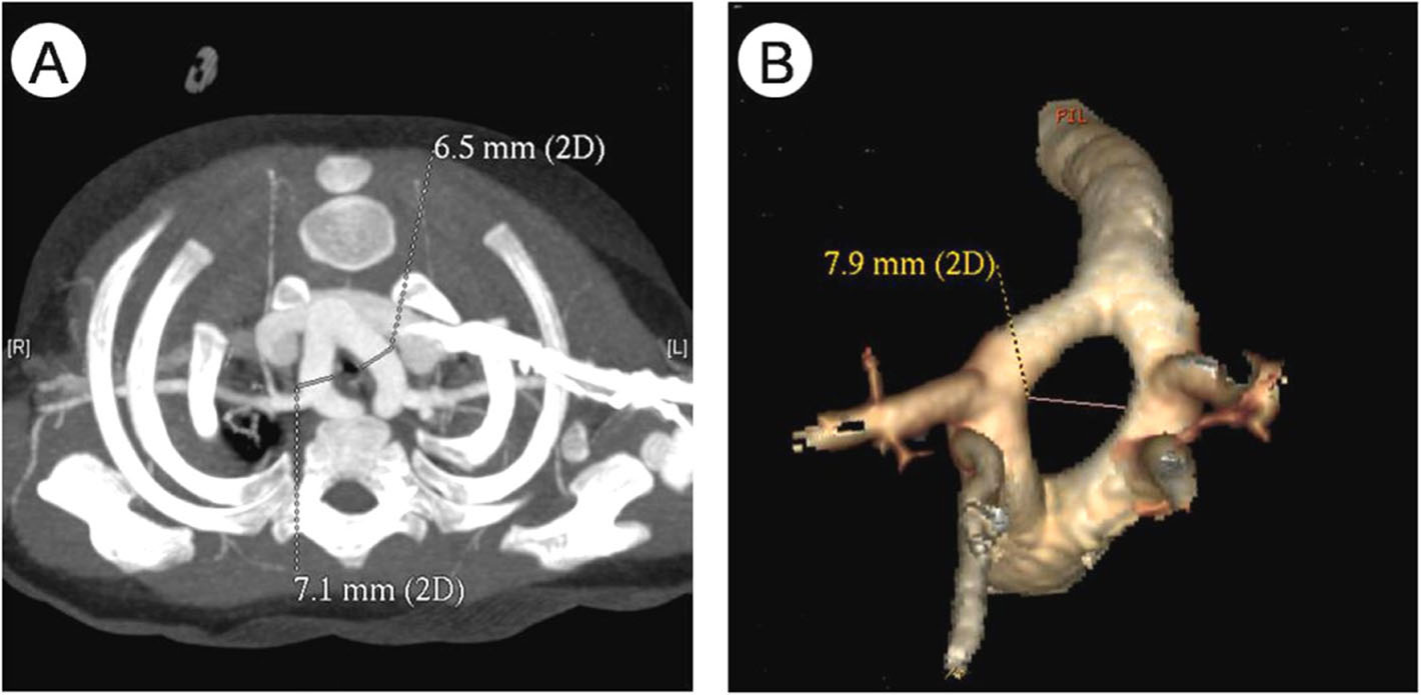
a:CT tomography of children with DAA b: Three-dimensional reconstruction of vascular rings of children with DAA
